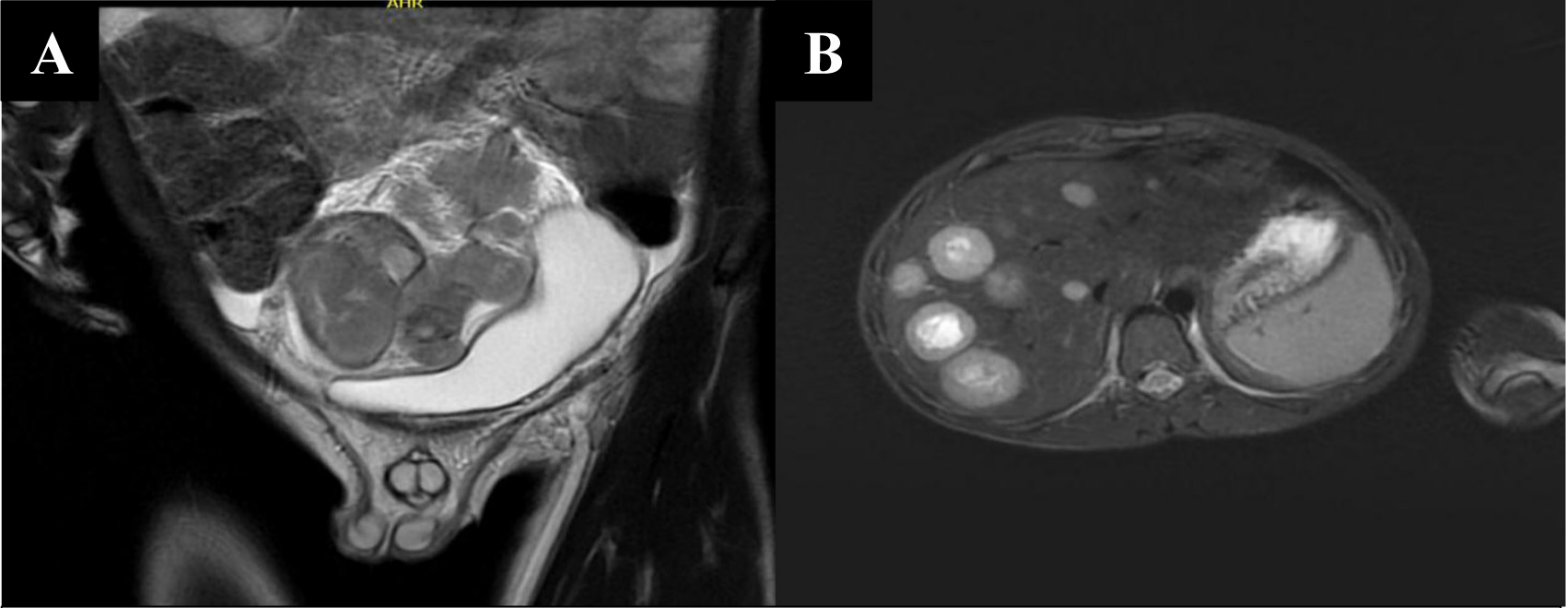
Coronal T2-weighted MRI image (A) shows bladder compression. Axial T2-weighted MRI image (B) shows multiple hepatic metastases with central necrosis.
